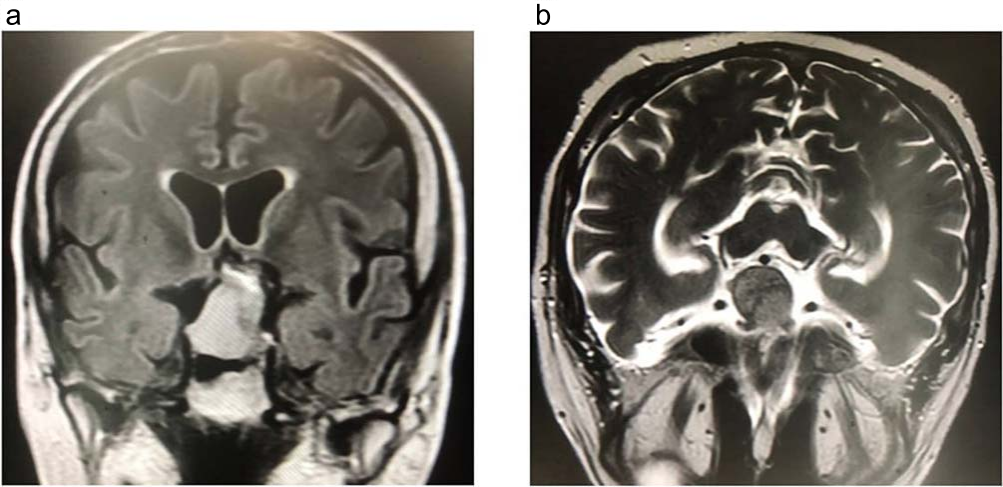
Brain MRI. (a) FLAIR coronal section. (b) T2WI axial section, show pituitary macroadenoma, measuring 35 × 27 × 25 mm. The sellar mass expands the sella and extends into suprasellar region, exhibiting a heterogeneous signal. It is in contact with optic chiasm and partially encases internal carotid artery (ICA).
